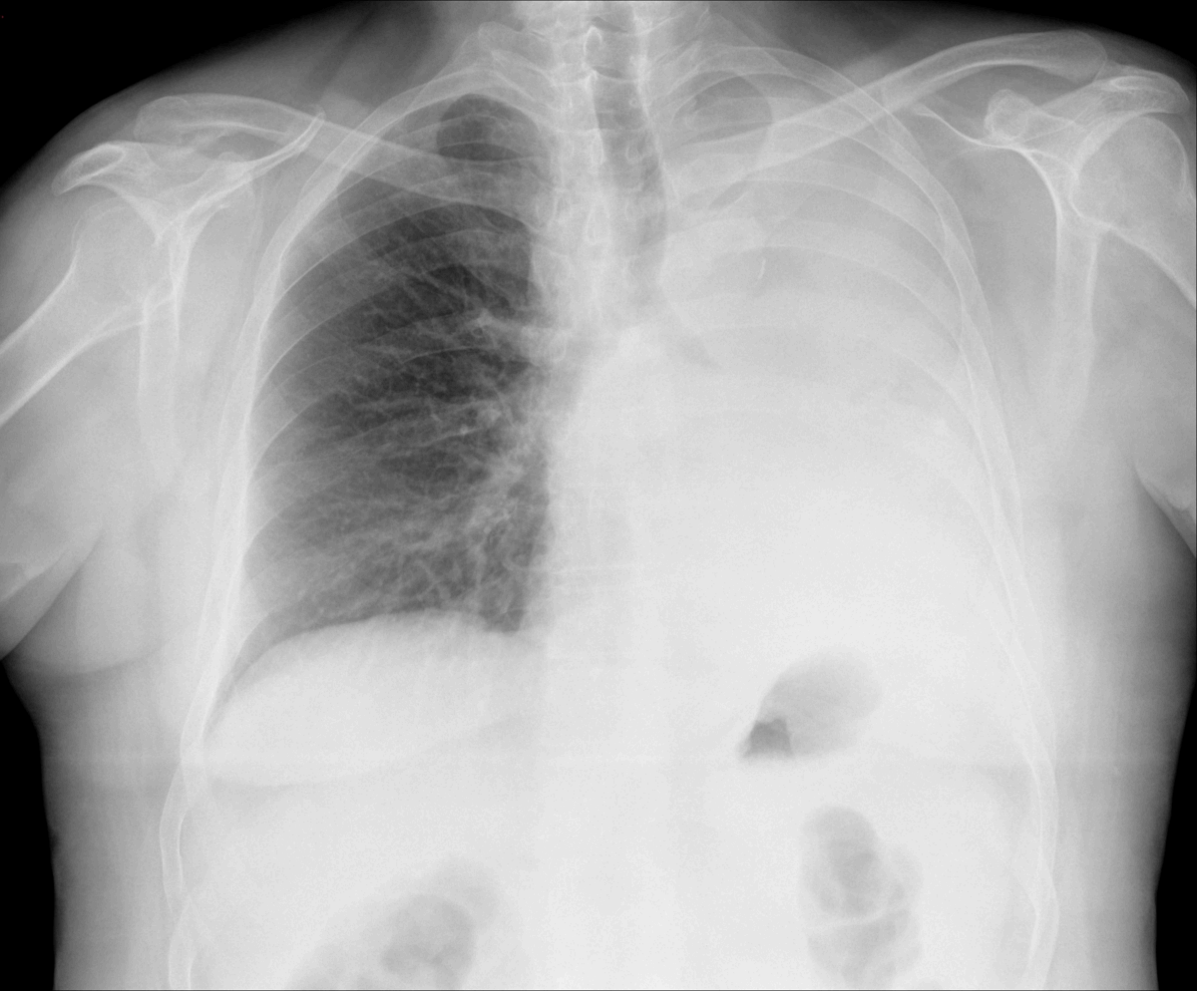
A chest x-ray corresponding to case number 3 shows complete opacification of the left hemithorax due to atelectasis of the left lung caused by bronchial carcinoma.
